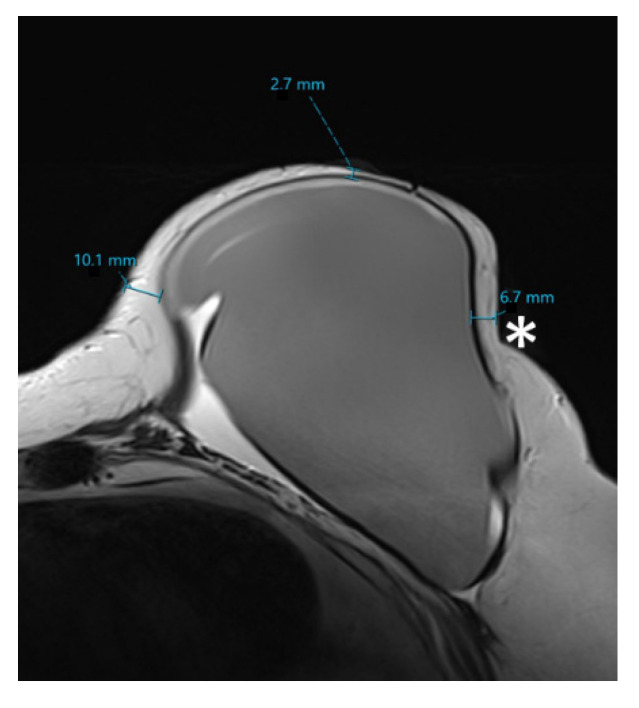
Example of compression (*) of the breast acquisition in T2-weighted image. Thickness measurements of retromamillary skin flap and at 3 and 9 o’clock. Measurements were performed using the integrated measurement tool of the Picture Archiving and Communication System (SECTRA AB, IDS7 Version 22.1, Linköping, Sweden).
